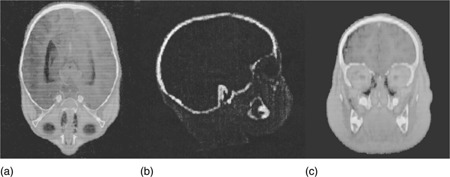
A transverse (a), sagittal (b), and coronal (c) view of a MR data set aligned to a CT set by one of the operators. The displayed bone comes from the CT, the soft tissue from the MR.
